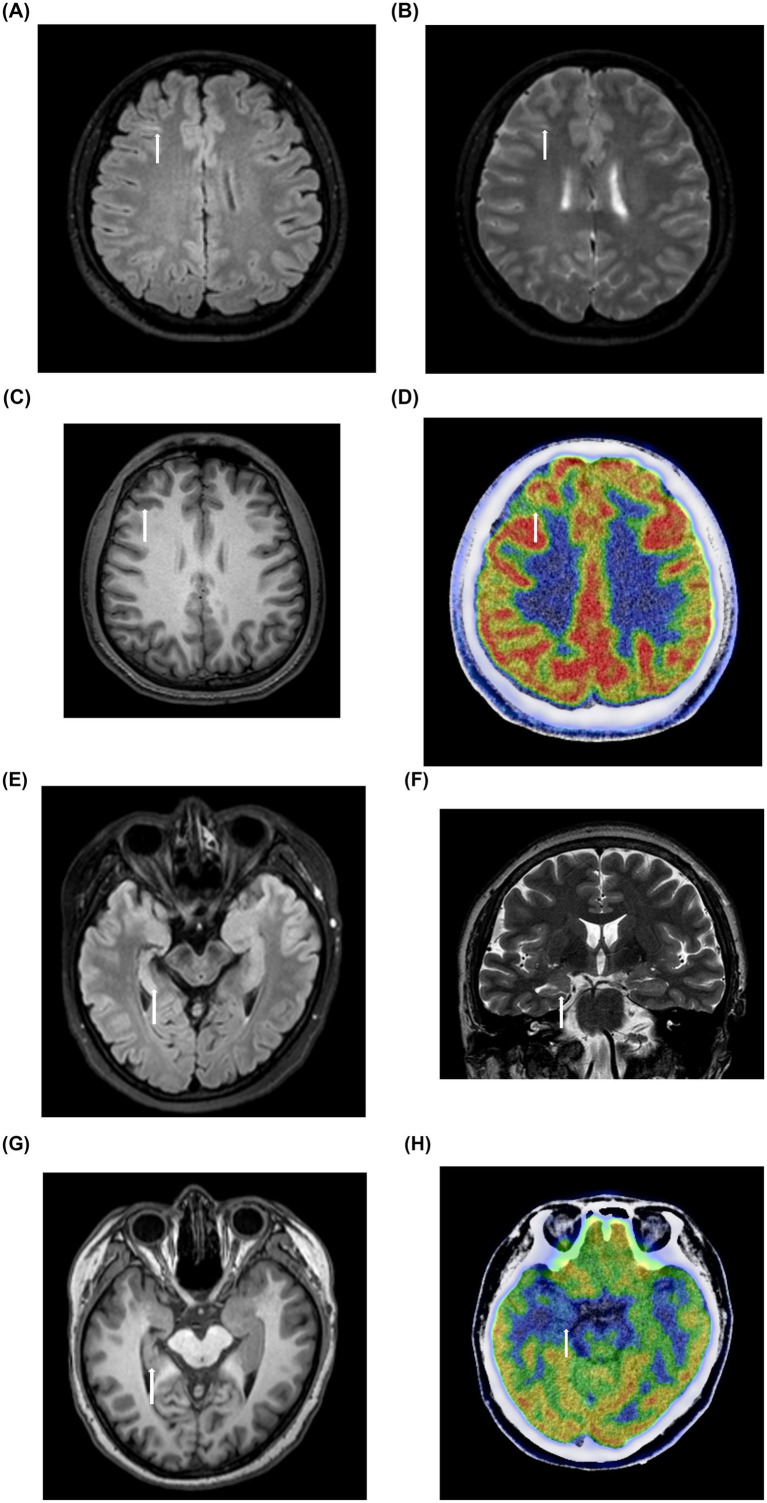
Case 1. FCDII type patient, accompanied by epilepsy for 5 years, with positive MR and PET manifestations. (A–C) Right middle frontal gyrus in axial and coronary FLAIR, T2WI, and T1WI in MRI showed abnormal signals (thick white arrow). (D) Hypometabolism of the right middle frontal gyrus in ^18^F-FDG PET (thick white arrow). Case 2. Right hippocampus sclerosis patient, with epilepsy for 2 years, (E–G) showed bilateral hippocampus in axial FLAIR, T2WI, and T1WI in MRI, showing abnormal signals. Right hippocampus was atrophy, and the signal was higher (thick white arrow). (H) Hypometabolism of the right hippocampus in ^18^F-FDG PET (thick white arrow).
